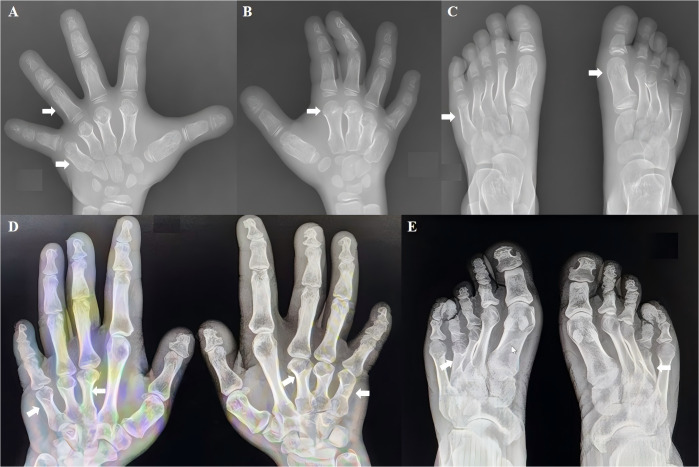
Radiographic features of *PTHLH*-related BDE2 in the proband and his mother. (A) Left hand radiograph of the proband demonstrating generalized shortening of the metacarpals and phalanges. (B) Evidence of premature epiphyseal fusion and metaphyseal widening in the proband. (C) Bilateral foot radiographs of the proband revealing diffuse shortening of the metatarsals and phalanges. (D) Bilateral hand radiographs of the mother showing marked shortening of metacarpals III–V and cone-shaped epiphyses. (E) Bilateral foot radiographs of the mother demonstrating metatarsal and phalangeal shortening.
